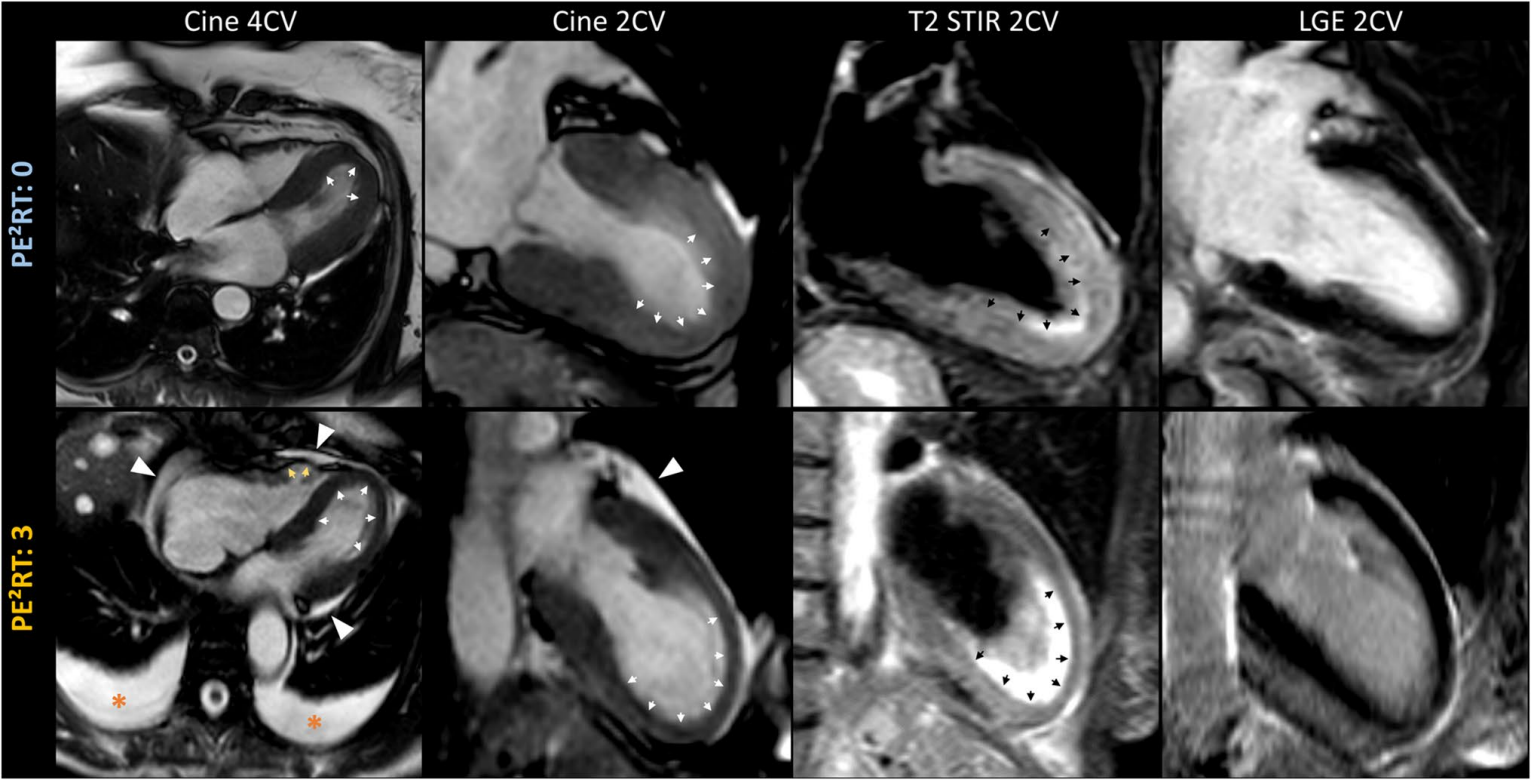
Representative cardiac magnetic resonance (CMR) imaging examples show two patients with typical diagnostic features of takotsubo syndrome (apical type). Regional wall motion abnormalities (apical ballooning) are visible on cine images in two- and four-chamber views (2CV, 4CV; white arrows) with corresponding myocardial oedema on T2-weighted short-tau inversion recovery images (T2 short-tau inversion recovery [STIR], black arrows) in the absence of late gadolinium enhancement (LGE). Images in the upper row show a 68-year-old female patient without major adverse cardiac event during follow-up (PE^2^RT score: 0; only slight pericardial fluid is visible on systolic cine images at the basal lateral wall, measuring  < 5 mm on cine images at diastolic phase). Images in the lower row show a 70-year-old female patient with the occurrence of a major adverse cardiac event during follow-up (PE^2^RT score: 3; moderate circumferential pericardial effusion  > 5 mm [white arrowheads], bilateral pleural effusions  > 20 mm [orange asterisks], and regional wall motion abnormalities involving the right ventricle [yellow arrows])
